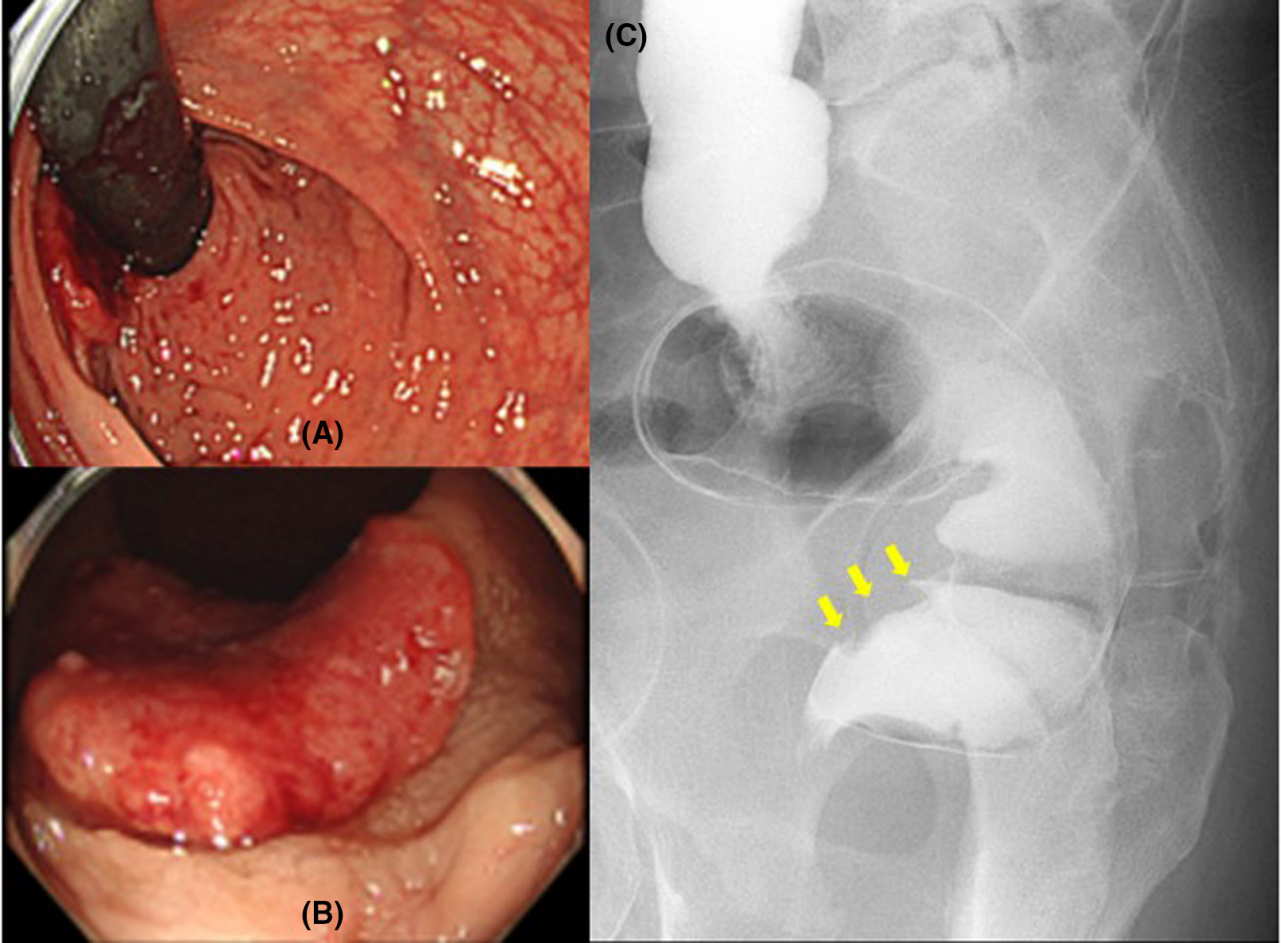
Preoperative findings. (A, B) Colonoscopy showing a mass lesion approximately 2 cm in diameter at the lower rectum (A: Looking up image, B: Front image). The degree of lesion invasion on endoscopic evaluation suggested infiltration into the deep submucosal layer. (C) Radiographic contrast enema image showing that the lower edge of the lesion was approximately 3 cm away from the dentate line (the lesion is in the area of the yellow arrows indicating the location of the lesion).
